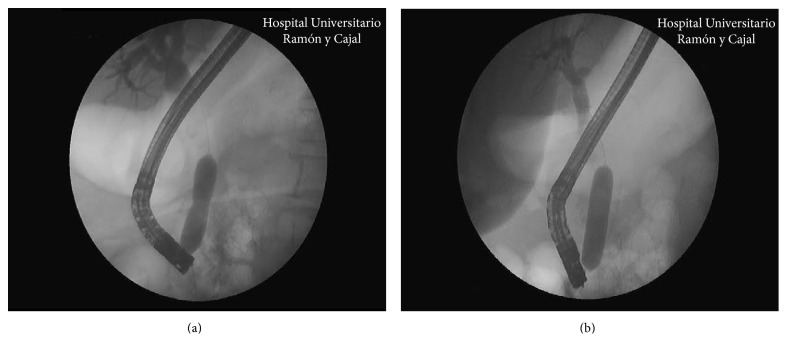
Partially (a) and totally (b) inflated balloon dilatation catheter on X-ray.
